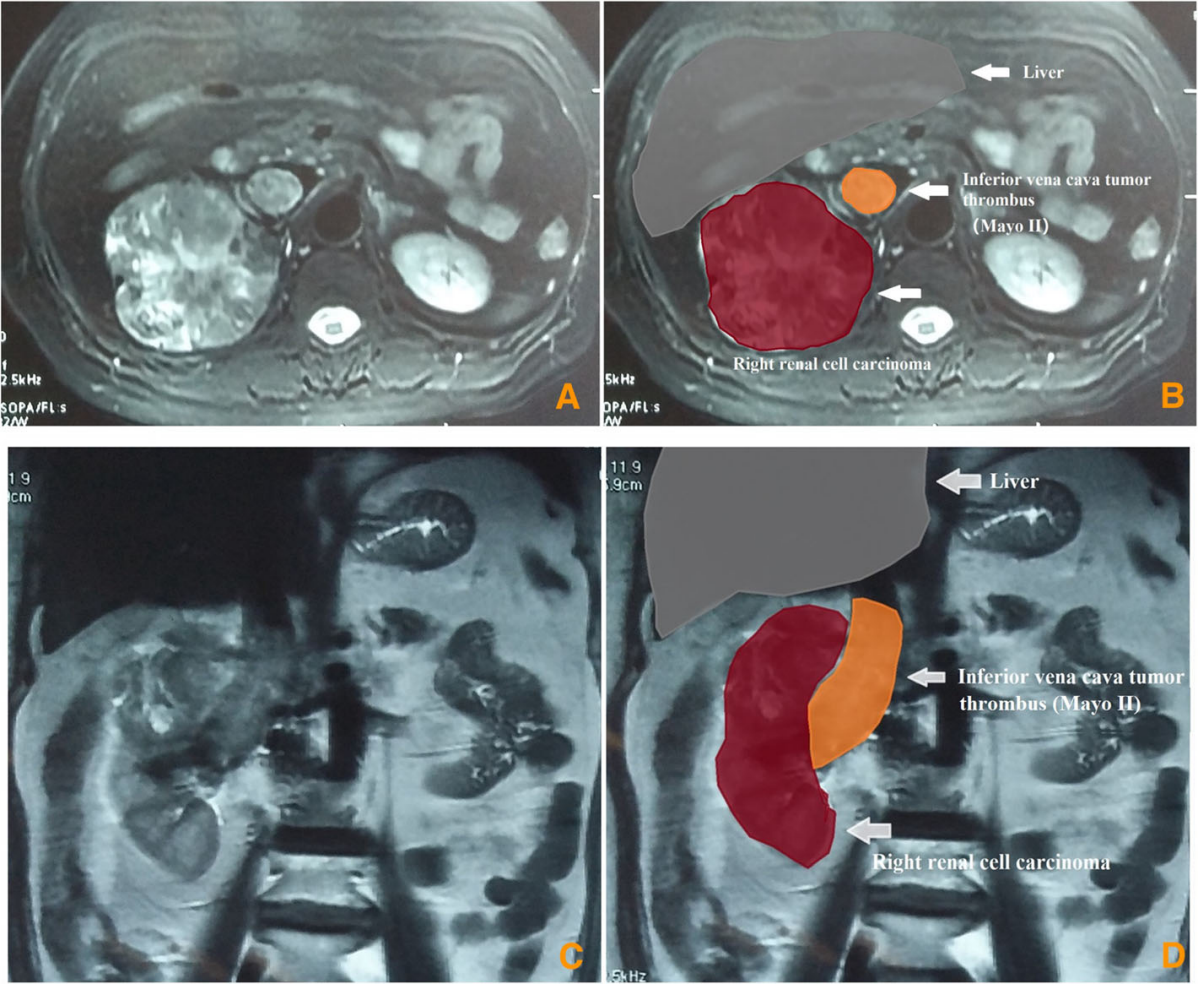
Renal tumor and inferior vena cava (IVC) tumor thrombus on the magnetic resonance imaging (MRI)
